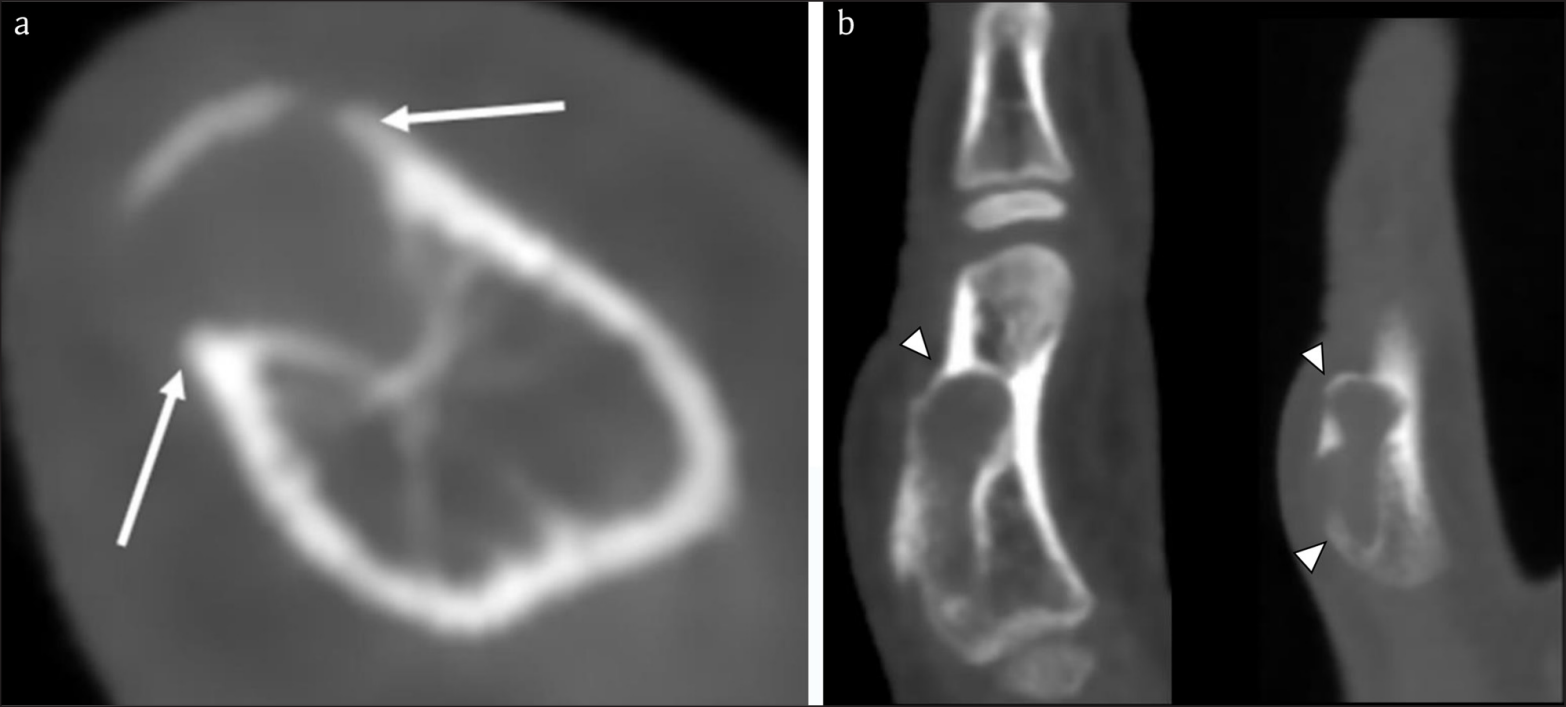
Case 1 – CBCT. Axial image (a). Sagittal reformatted images (2 adjacent slices) (b). Note an expansile radiolucent lesion at the dorsal phalangeal cortex with sclerotic borders (arrows in a). The smaller intramedullary and a larger exophytic components of the lesion are clearly depicted. The cortex is thinned and partially destructed with overhanging edges (arrowheads in b).
